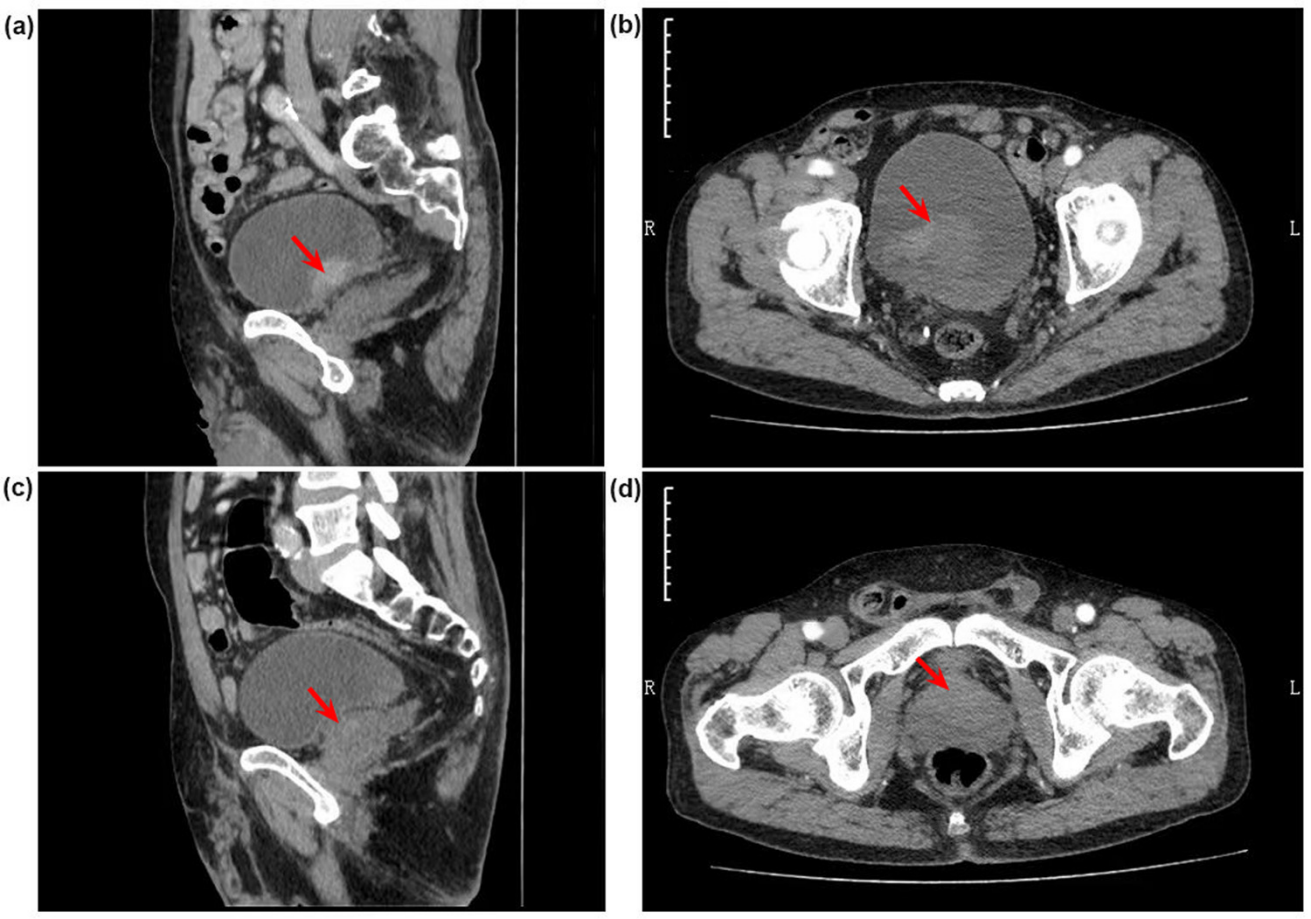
CT scan of the pelvic cavity in August 2020. (a and b) Show the bladder carcinoma: a soft tissue mass (about 38 mm × 23 mm) with a wide base was seen in the bladder triangle, and the mass was uniformly enhanced in contrast CT. The bladder wall shown with limited thickening and stiffness. (c and d) Show the prostate carcinoma: The prostate was enlarged (about 52 mm × 43 mm) with uniform density. (a and c) belongs to the sagittal position. (b and d) belongs to the axial position.
